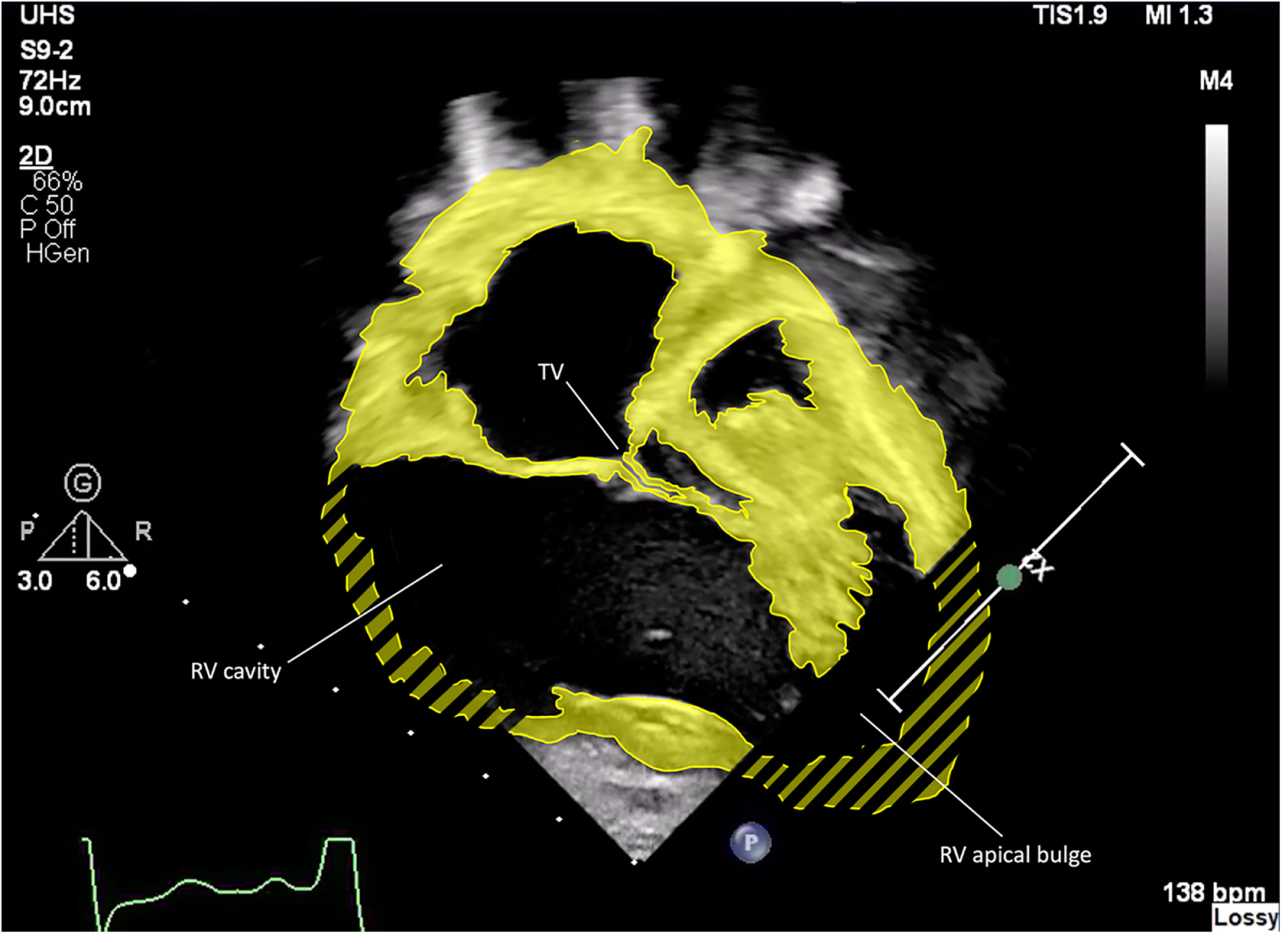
Enhanced 2D echocardiographic subcostal view. Note the RV apical bulge encircling the hypoplastic LV.
